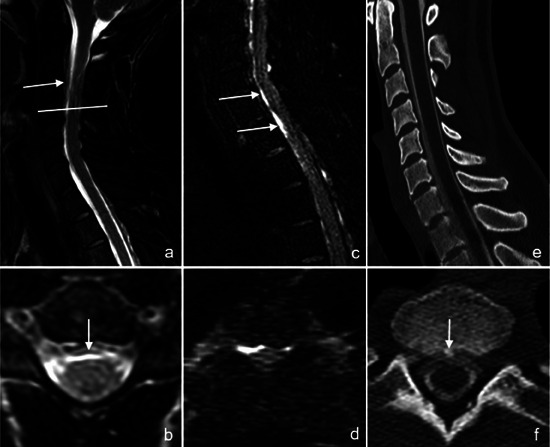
Presentation of a case in which the ventral predominance of a cerebrospinal fluid (CSF) lamella is better visualized on contrast‐enhanced heavily T2‐weighted fluid‐attenuated inversion recovery (ceHT2‐FLAIR) MRI than on noncontrast MR myelography. A 43‐year‐old female patient with intracranial hypotension (case #2) presented with therapy‐resistant, position‐dependent headache for 4 weeks without any history of trauma. On noncontrast MRI, the modified DIXON T2 sequence revealed a right lateral and ventral epidural fluid lamella in the sagittal plane (white arrow) (a), which was confirmed in the axial plane (white arrow) (b). On ceHT2‐FLAIR, the epidural CSF lamella is clearly visible despite motion artifacts and demonstrates strong contrast enhancement in both sagittal and axial planes immediately postinjection (white arrows) (c, d) with a ventral predominance. CT myelography in the sagittal and axial plane (e, f) confirmed the presence of the CSF lamella, and a causative osteophyte (white arrow) could be identified. The patient therefore received a targeted epidural blood patch. In this case, ceHT2‐FLAIR confirmed the findings of noncontrast MR myelography and visualized the predominance of a ventral CSF lamella even better.
